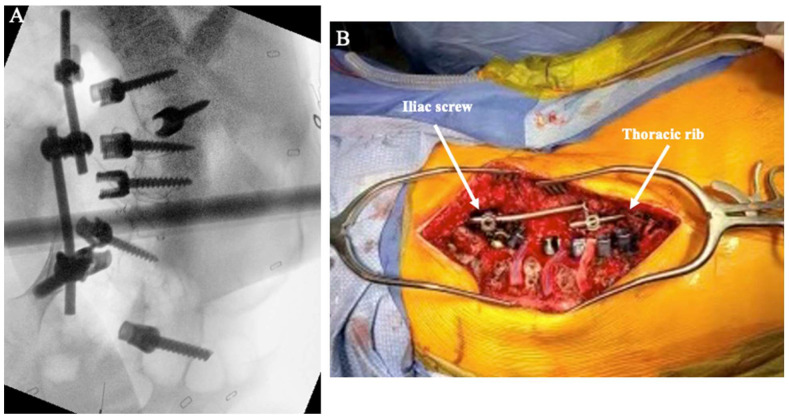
Intraoperative X-ray showing a construct-to-construct internal distraction technique with an iliac screw serving as the distal anchor point and the last thoracic rib serving as the proximal anchor point (A). Intraoperative photo showing the iliac screw to thoracic rib setup before distraction is performed (B).
